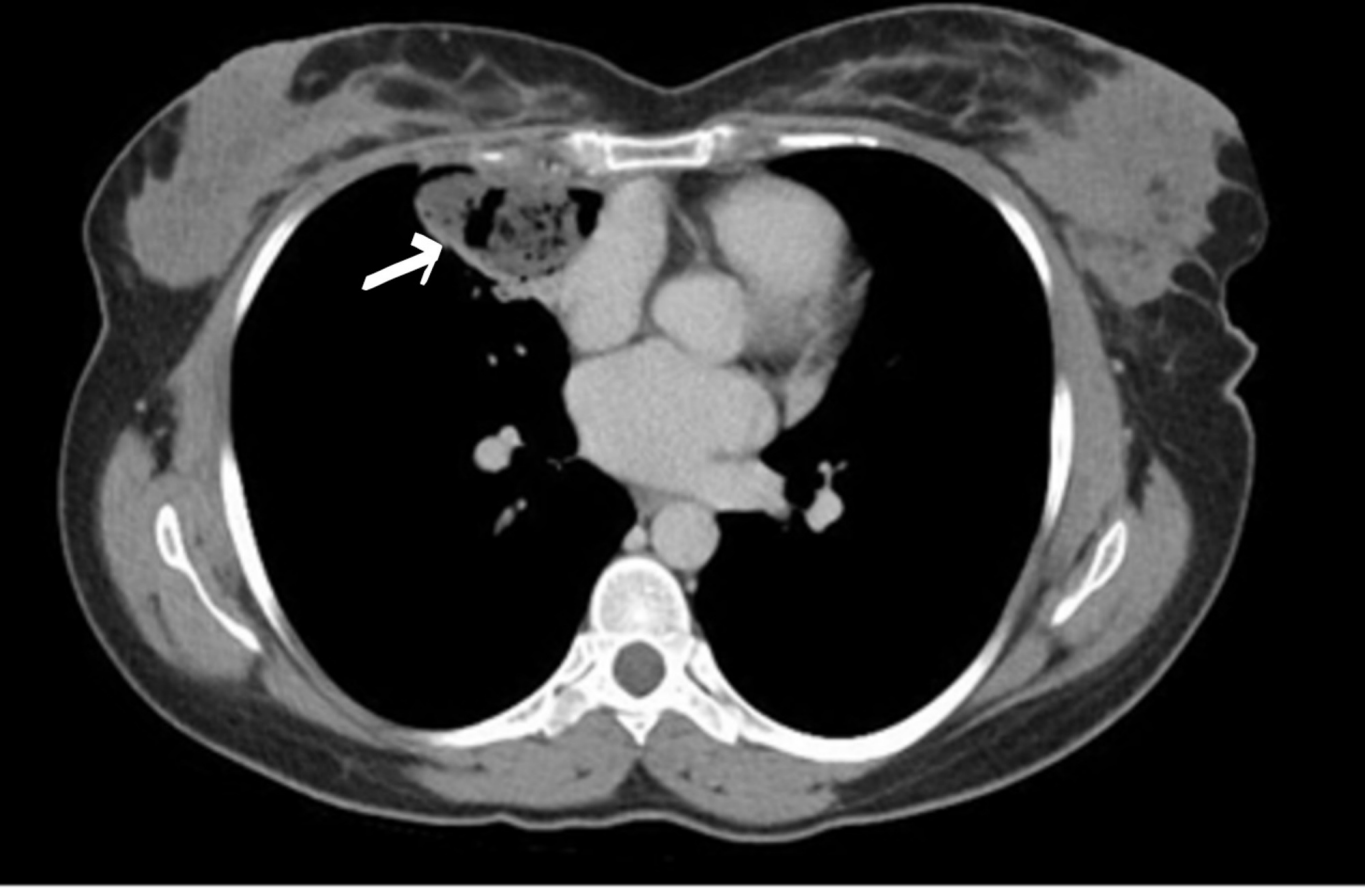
Computed tomography (CT) of the chest revealed a cystic mass in the right upper lung zone
